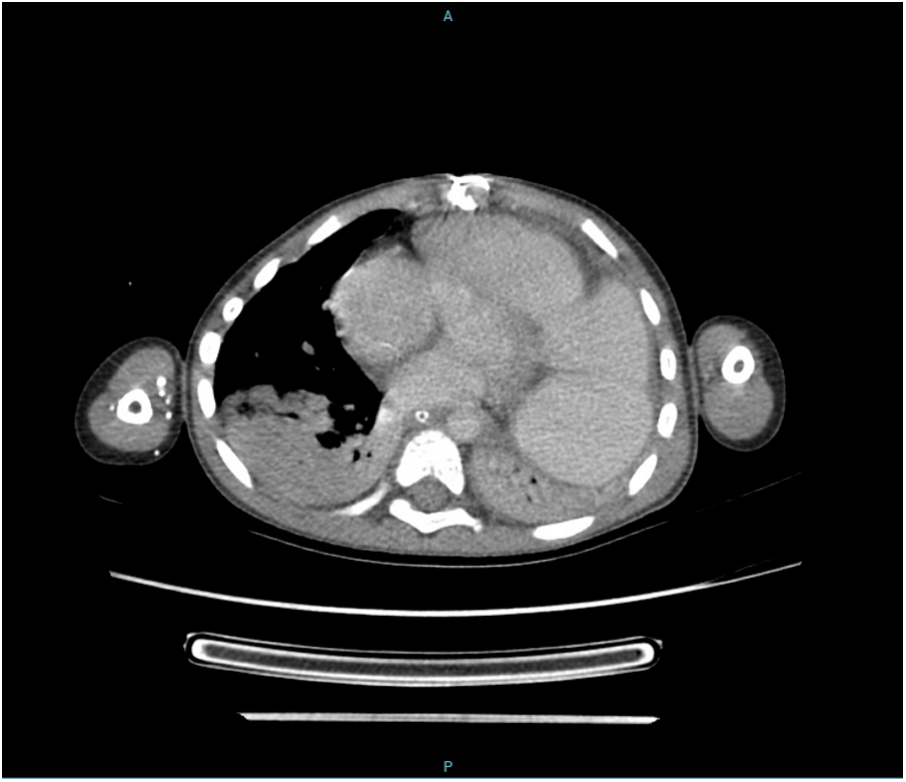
CT scan showing dilation of the RV-PA conduit (and also right and left lower lobes atelectasis).
